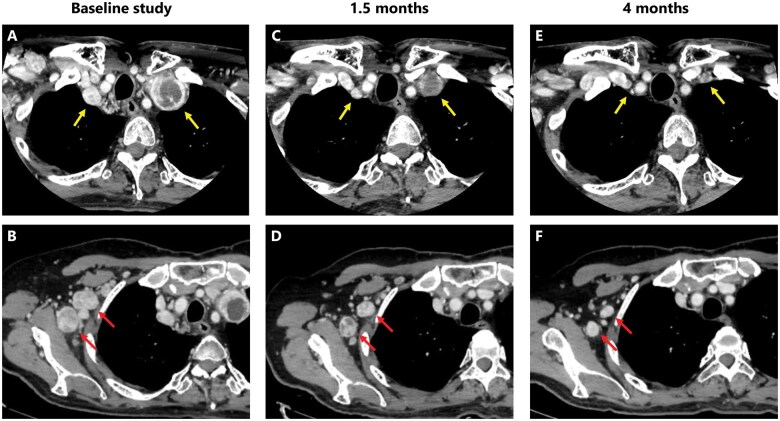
Representative axial, enhanced computed tomography pictures of cervical and axillary lymph node metastases (arrows) taken just before (A, B), 1.5 months after (C, D), and 4 months after (E, F) commencement of selpercatinib. Computed tomography imaging findings of metastases in the patient treated with selpercatinib, with subpanels labelled from A to F.
